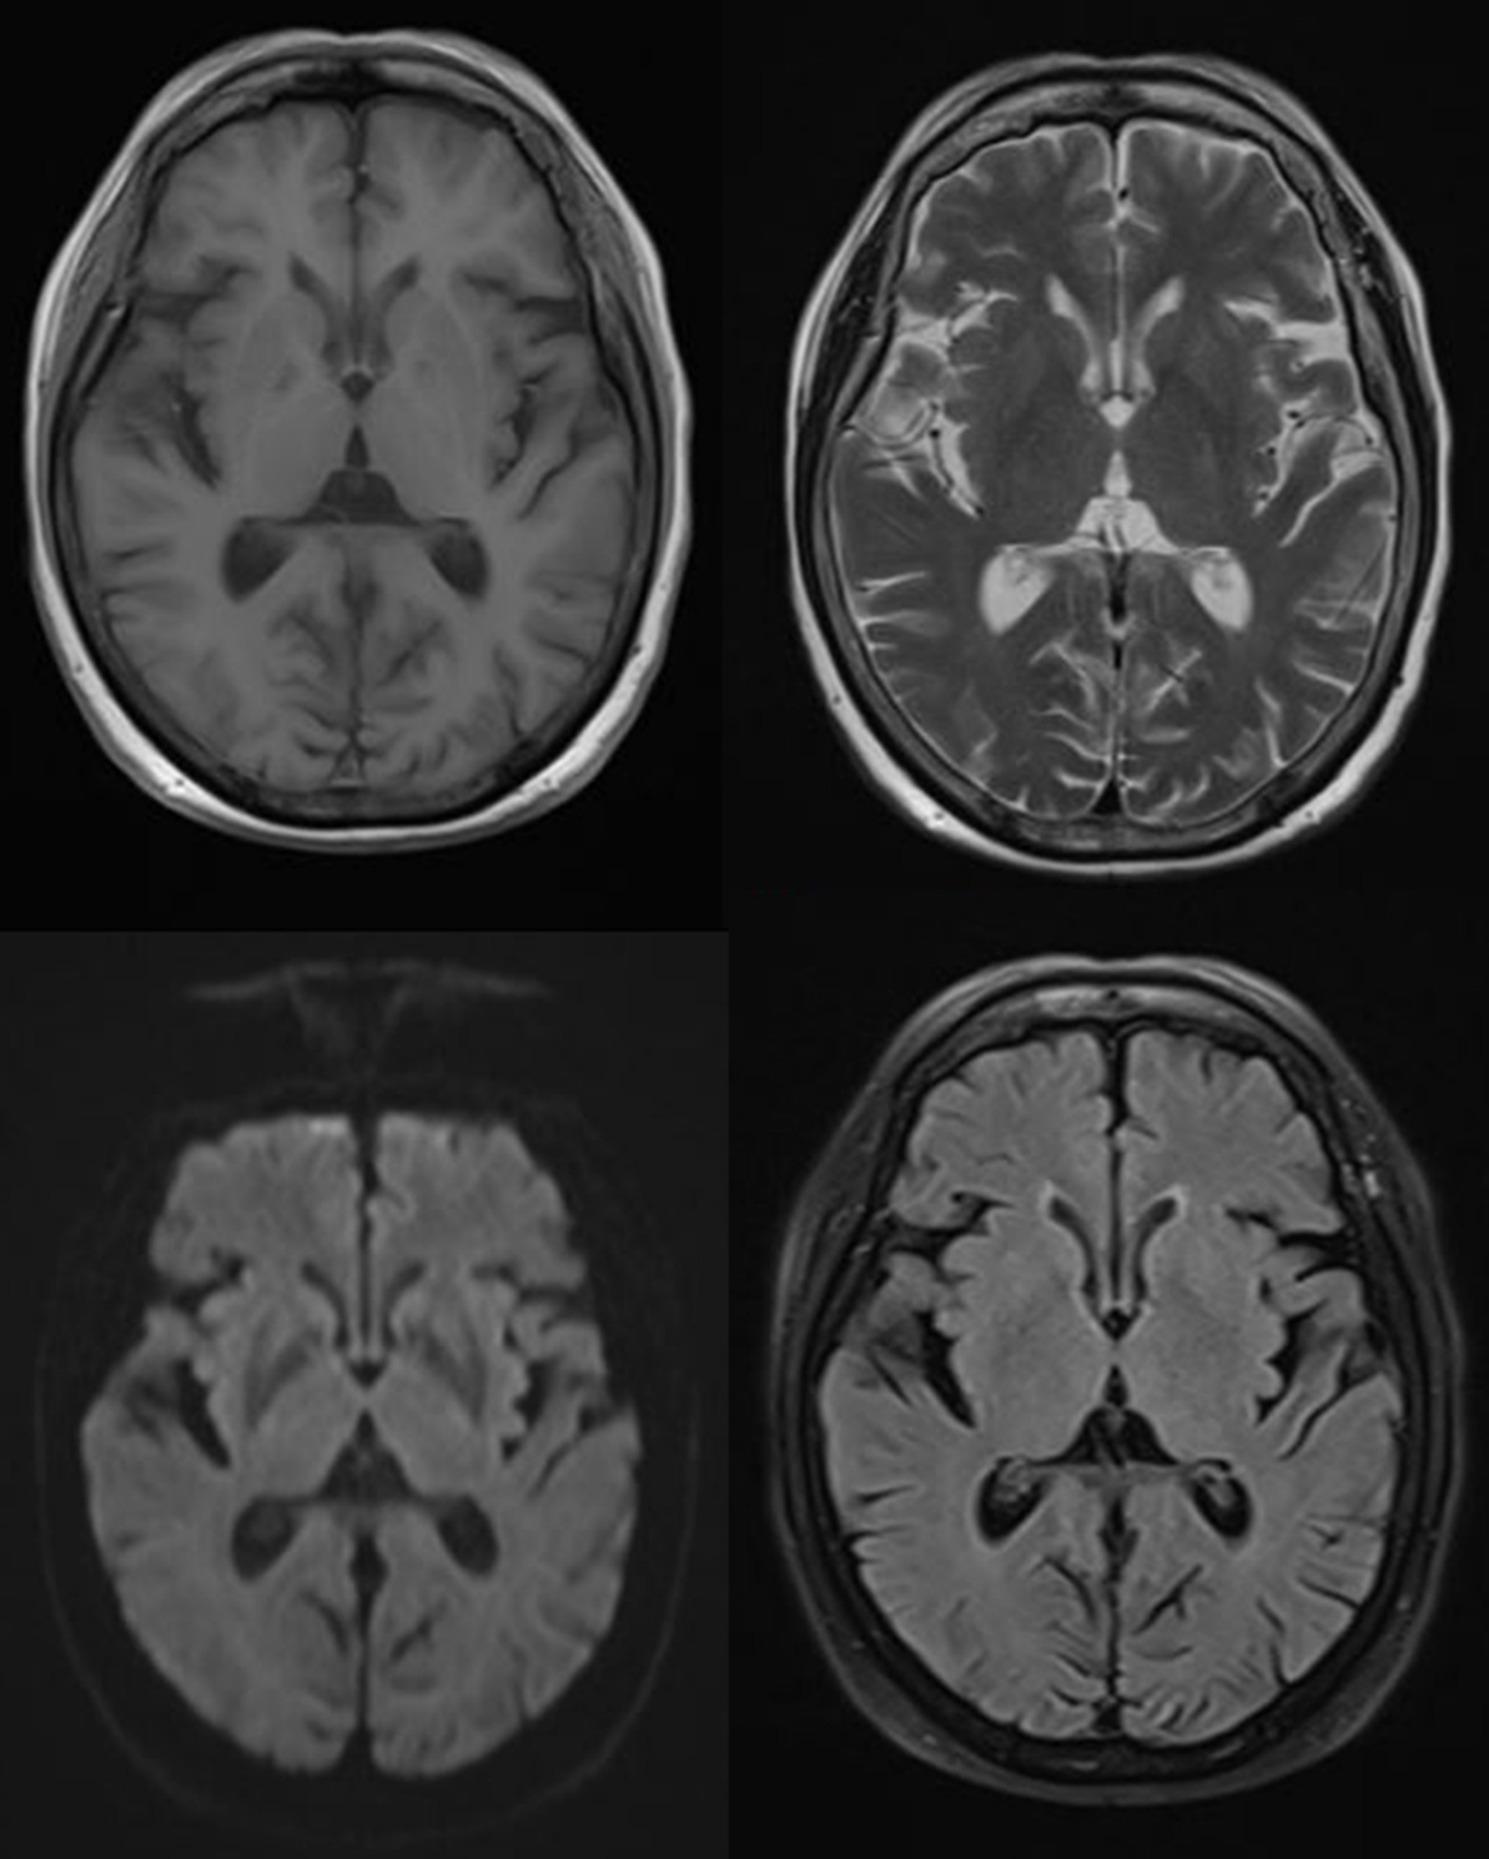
Neuroimaging findings showing T1WI, T2WI, DWI, and FLAIR sequences
